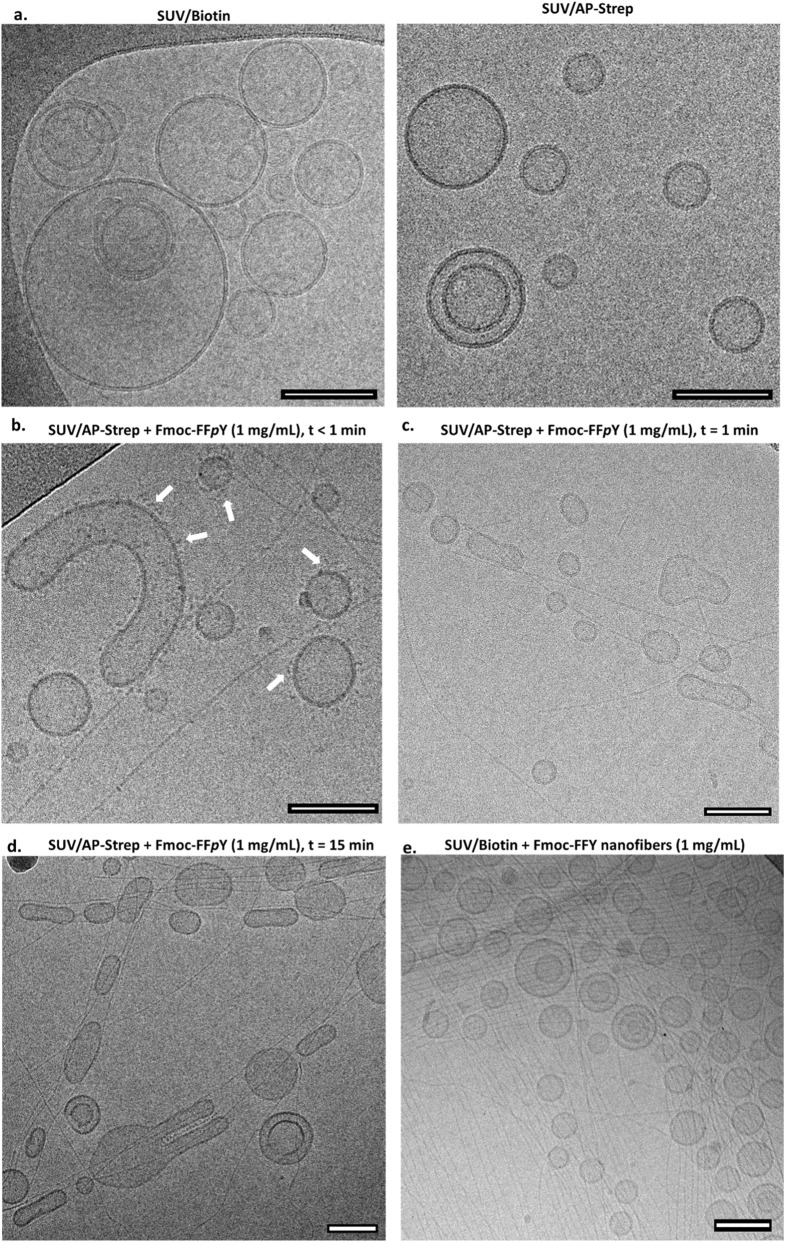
Typical cryo-TEM images of (a) SUV/Biotin (left) and SUV/AP-Strep (right), SUV/AP-Strep in presence of Fmoc-FF*p*Y observed (b) less than 1 min, (c) after 1 min and (d) 15 min later. The white arrows show the presence of small aggregates. (e) SUV/Biotin brought in contact during 24 h with Fmoc-FFY self-assembled nanofibers formed by annealling (More et al., 2024). The error bar indicates a length of 100 nm.
